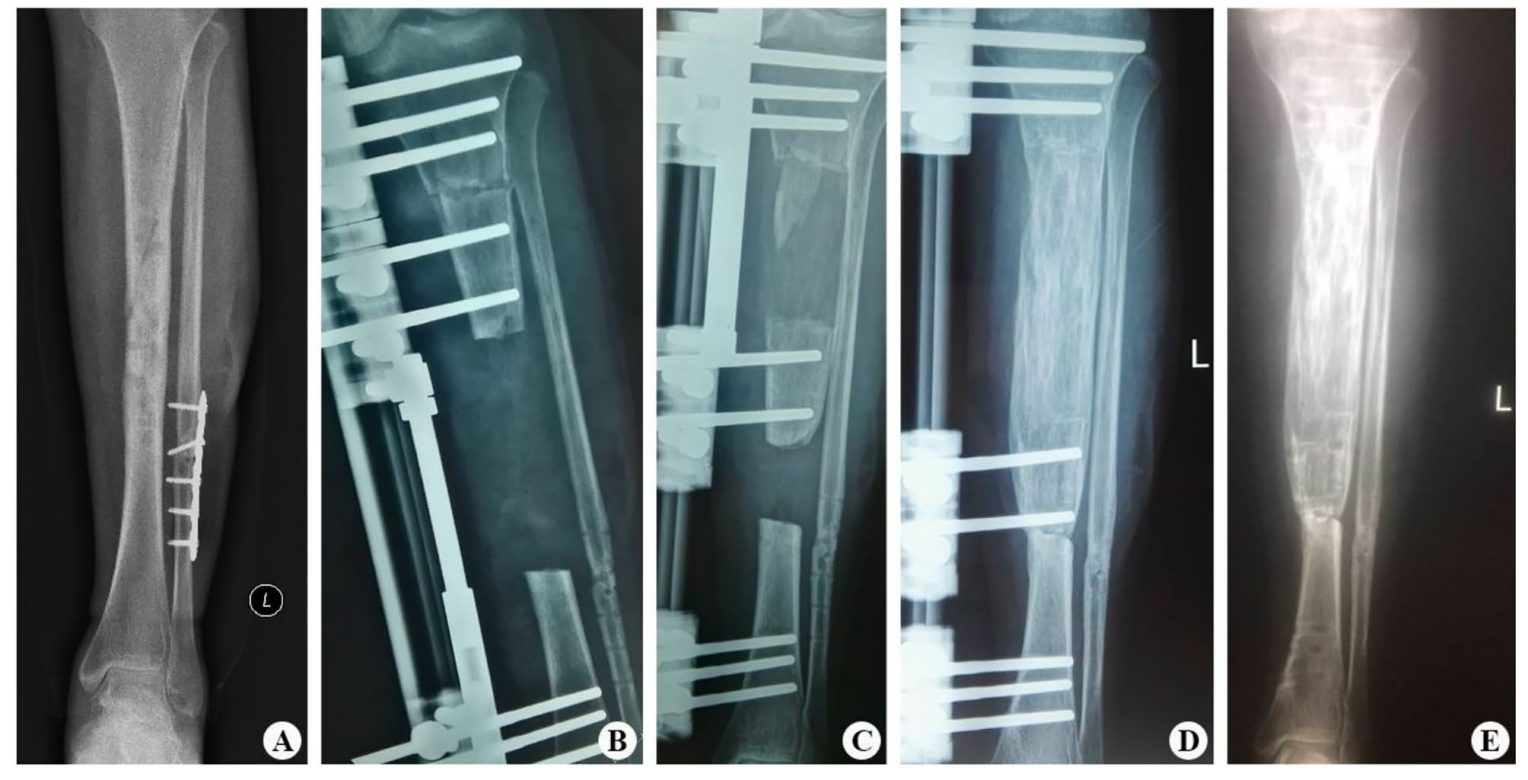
A 47-year-old female with post-traumatic osteomyelitis of the left tibia was treated with single-level bone transport using Orthofix external fixator. (A) X-ray of left tibia before single-level bone transport surgery. (B) Postoperative X-ray of the left tibia showed that DS after debridement was almost 7.4 cm. (C) X-ray at 4 weeks in the distraction phase. (D) Bone transport was completed with good consolidation, and docking union was achieved in the 9th postoperative month. (E) The external frame was removed with an excellent bone result after 11 postoperative months
